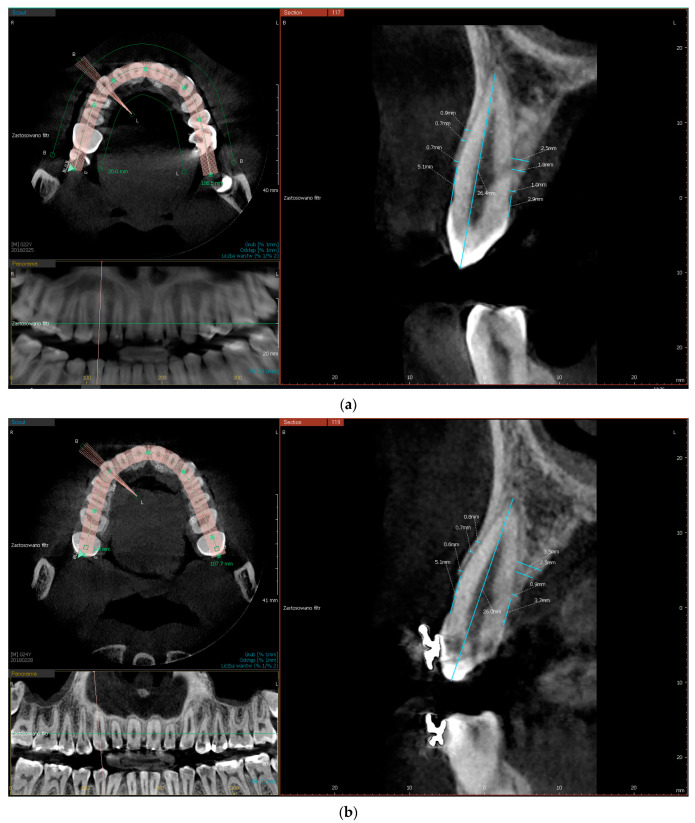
(a) CBCT scan of tooth No. 13 taken before treatment. CEJ-B = 5.1 mm, CEJ-P = 2.9, buccal plate thickness 0.5 mm = 0.7 mm, buccal plate thickness 3.5 mm = 0.7 mm, buccal plate thickness 5 mm = 0.9 mm 2.9 mm, palatal plate thickness 0.5 mm = 1 mm, palatal plate thickness 3.5 mm = 1.8 mm, palatal plate thickness 5 mm = 2.5 mm. (b) CBCT scan of tooth No. 13 taken after treatment. CEJ-B = 5.1 mm, CEJ-P = 3.7 mm, buccal plate thickness 0.5 mm = 0.6 mm, buccal plate thickness 3.5 mm = 0.7 mm, buccal plate thickness 5 mm = 0.8 mm, palatal plate thickness 0.5 mm = 0.9 mm, palatal plate thickness 3.5 mm = 2.5 mm, palatal plate thickness 5 mm = 3.5 mm.
